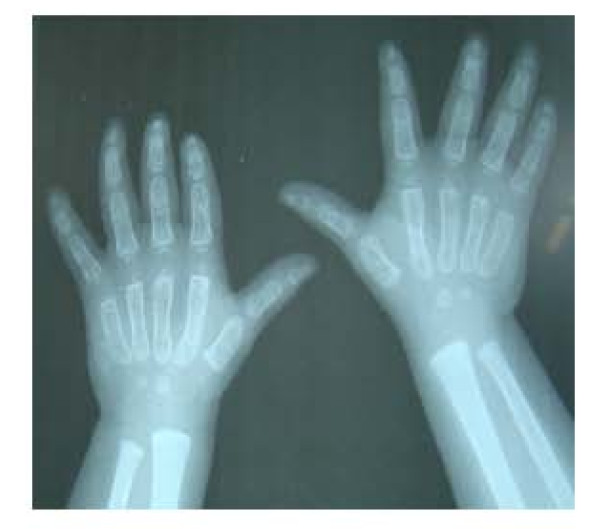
X-ray hands show evidence of osteopenia and uneven fingers.
